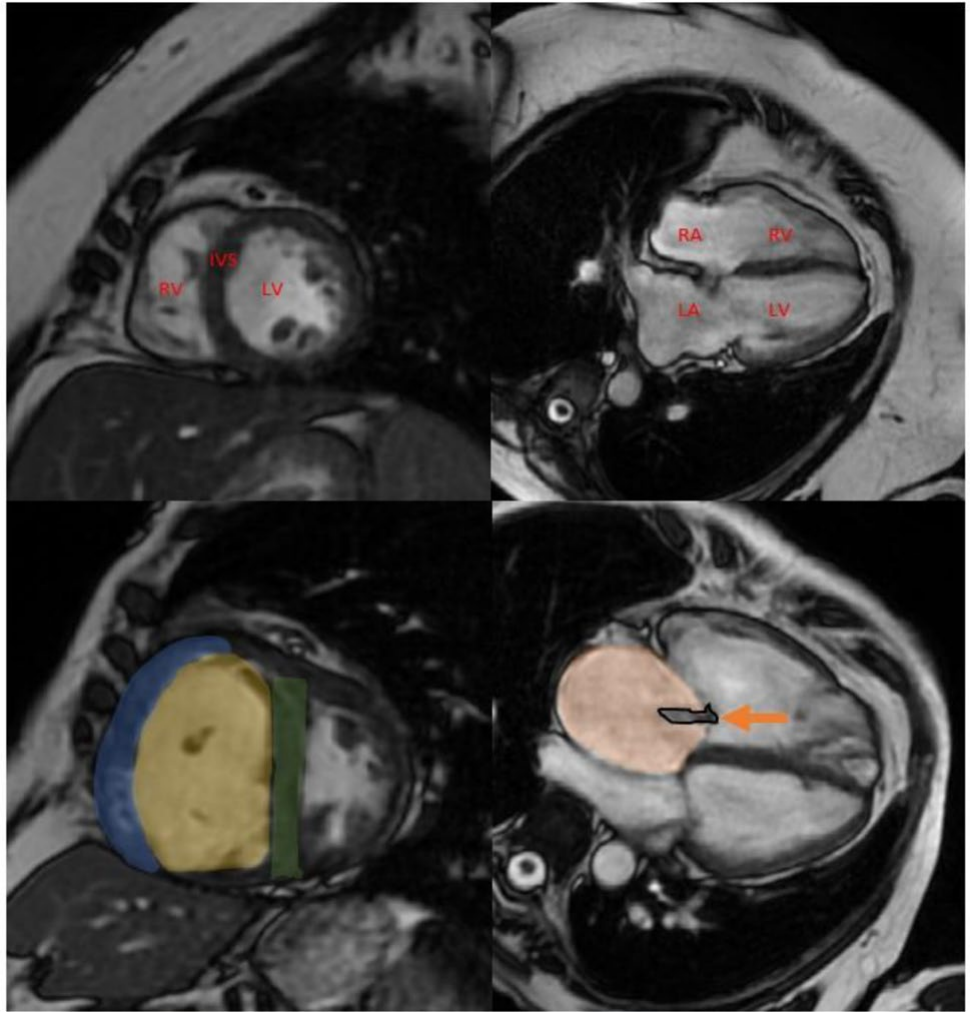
Cardiac MRI cine images (short-axis: left, four chamber: right). *Top row:* normal MRI. RV; right ventricle, LV; left ventricle, IVS; interventricular septum, RA; right atrium, LA; left atrium. *Bottom row:* pulmonary arterial hypertension features including a hypertrophied RV myocardium (blue), dilated RV chamber (yellow), IVS straightening (green), RA enlargement (orange) and tricuspid regurgitation jet (arrow)
